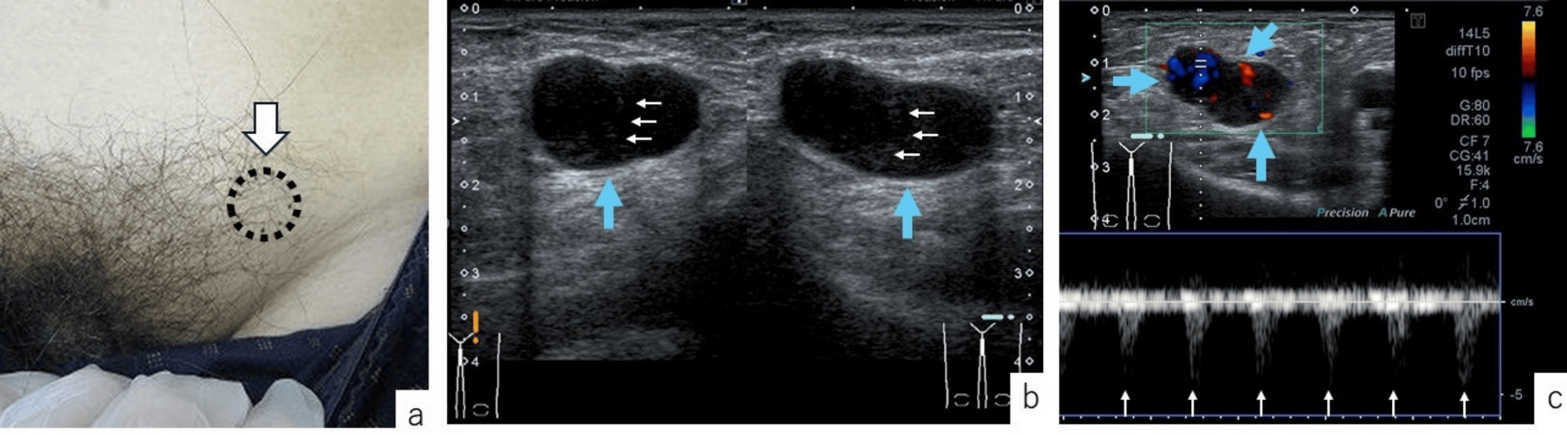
Preoperative Findings a. Initial examination findings: A painless, elastic, firm tumor approximately 25×15 mm in size (arrow) was palpable slightly cranial and medial to the left inguinal region. The tumor was not adherent to the skin or underlying tissue, and the overlying skin appeared normal. b. Findings from surface ultrasonography: Short- (left) and long-axis (right) views showed a hypoechoic tumor (blue arrows), approximately 26×14 mm in size, with septum-like structures within (white arrows), located in the subcutaneous fat layer. c. Doppler ultrasonography findings: Abundant blood flow from various directions was observed within the tumor (blue arrows), along with a regular pulsatile waveform (white arrows).
